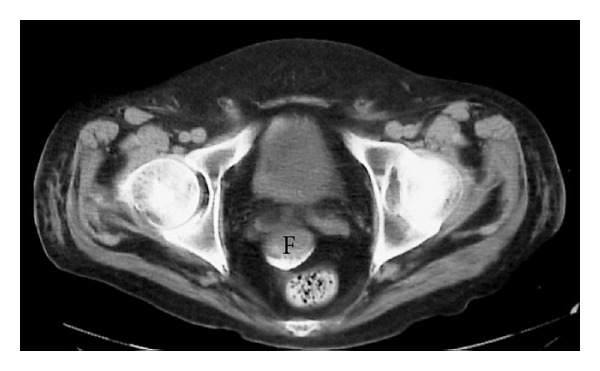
CT scan through the pelvis at the level of the acetabula demonstrates fluid (F) between the seminal vesicles and the rectum. Dense barium is present dependently within the fluid.
